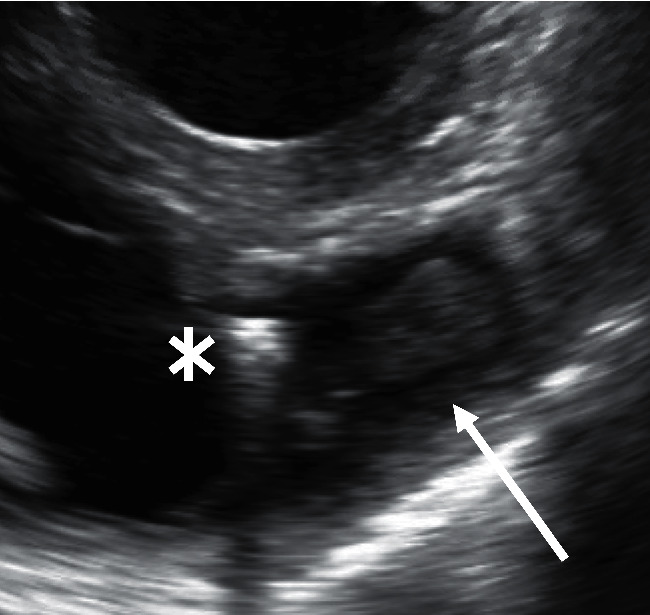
Transesophageal echocardiography image of Micra device (asterisk) with attached vegetation (arrow).
